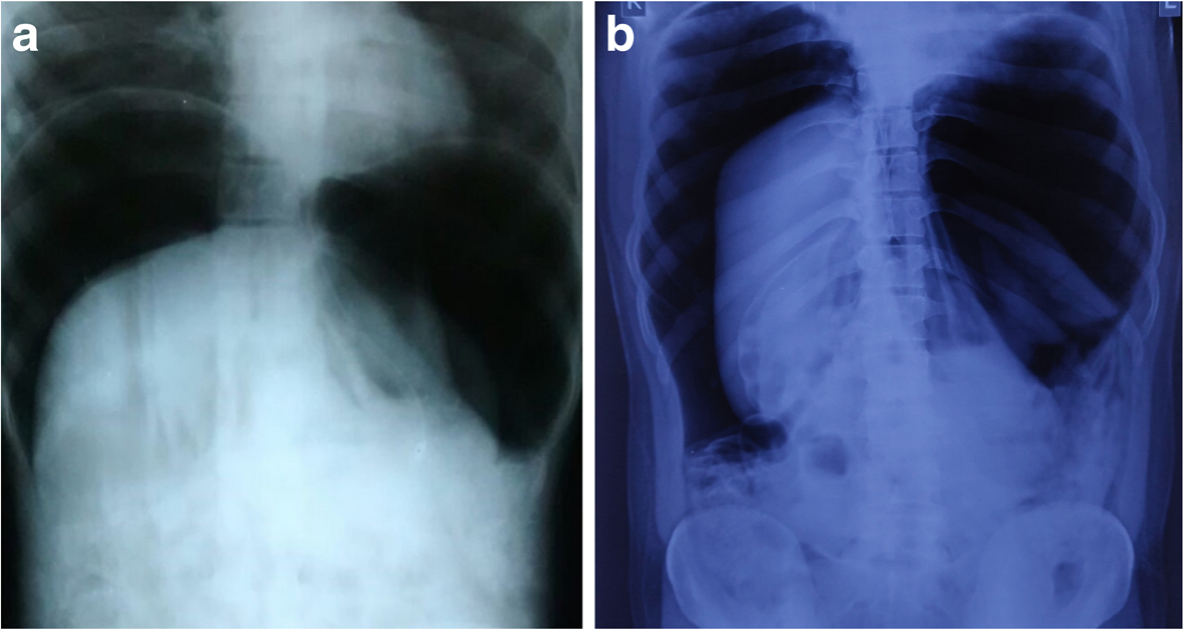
a and b Abdominal x-ray showing gross free air in peritoneal cavity
